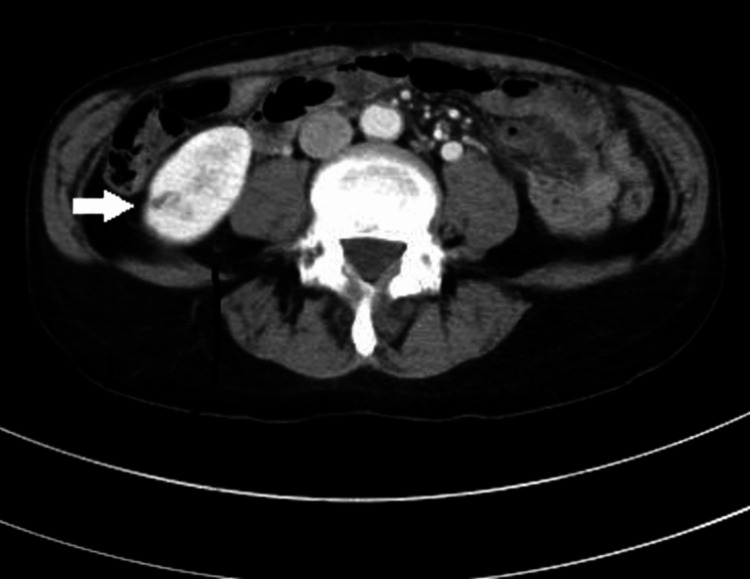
Contrast-enhanced abdominal CT image A small infarction was observed in the right kidney (arrow).
